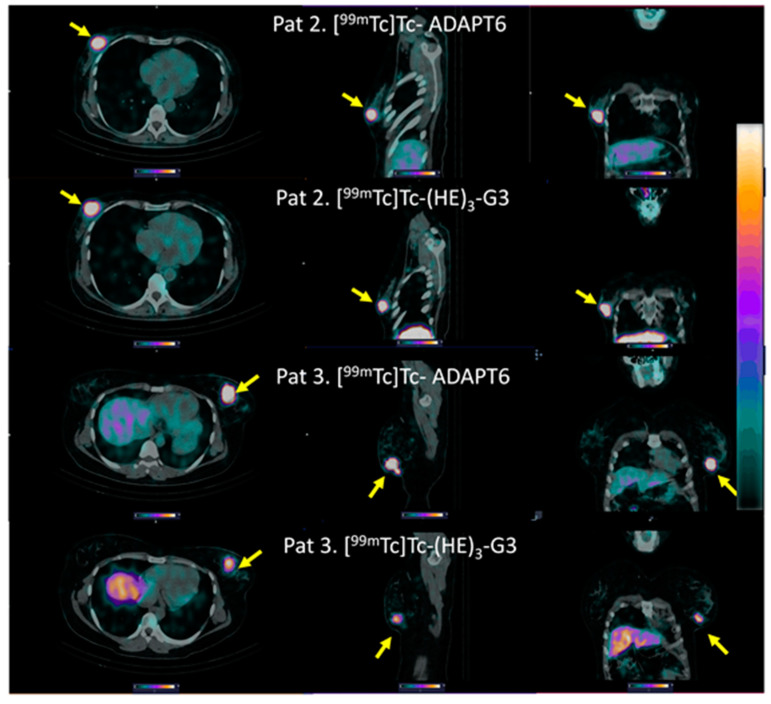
Representative SPECT/CT images of primary HER2-positive tumours after injection of [99mTc]Tc-ADAPT6 (2 h after injection) and [99mTc]Tc-(HE)_3_-G3 (4 h after injection). Arrows point at tumours. The upper setting of a linear intensity scale is adjusted to SUV 6.8 in all images.
